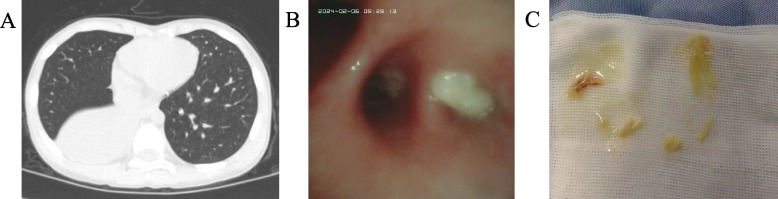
The discovery of radiological examination and electronic bronchoscopy. A This was an image of a 7-year-old boy's chest CT scan upon admission. The chest CT showed large areas of consolidation. B Electronic bronchoscopy revealed phlegm clots blocking the bronchus. C The plastic cast blocking the bronchus was removed
